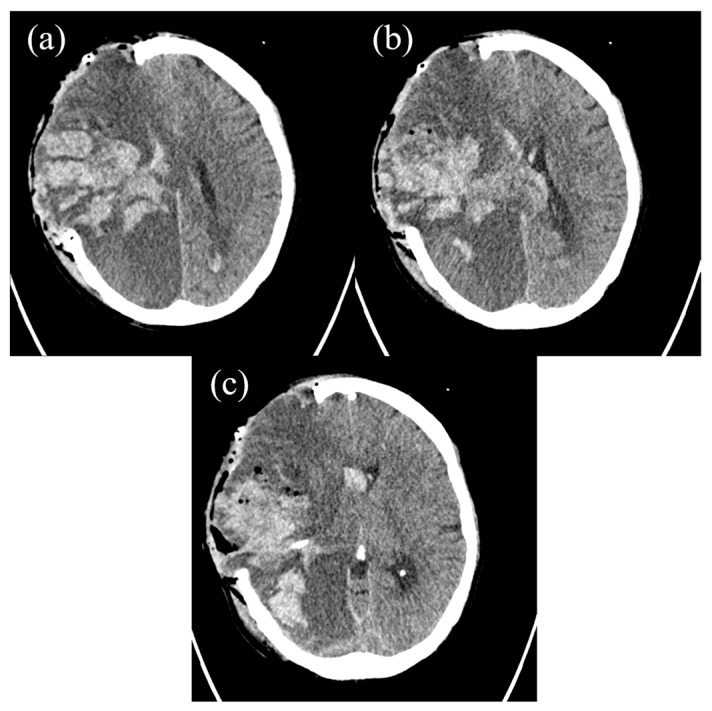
(a–c) The postoperative axial cranial CT scan revealing further ICH in the right frontal and parietal lobes.
